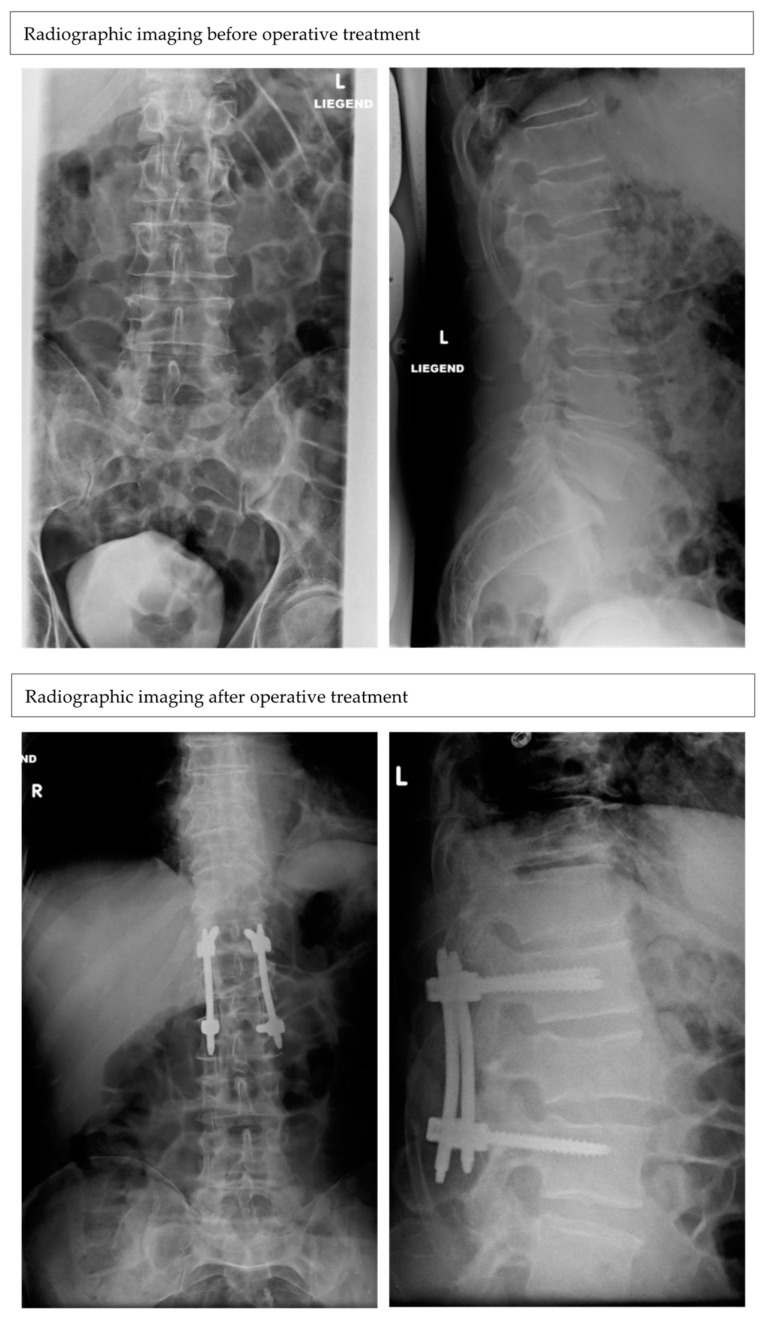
Radiographs (anterior–posterior and lateral) of a patient, 62 years old, with an A3 fracture of L1 after a car crash, before and after operative treatment with a dorsal stabilization. Postoperative reduction was still present after the last follow-up.
